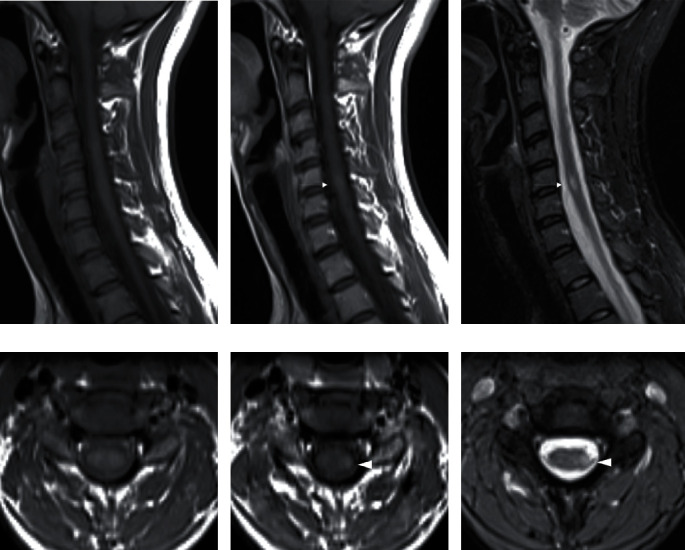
Cervical spinal cord MRI of an MS patient in our center, a 29-year-old female with RRMS: (a) sagittal unenhanced T1WI, (b) contrast-enhanced T1WI, and (c) T2WI TIRM revealing a short-segment, mildly contrast-enhancing spinal cord lesion at the level of C5-6 (arrows). Corresponding axial images showing the same lesion (arrowheads) on (d) unenhanced T1WI, (e) contrast-enhanced T1WI and (f) T2WI TIRM.
